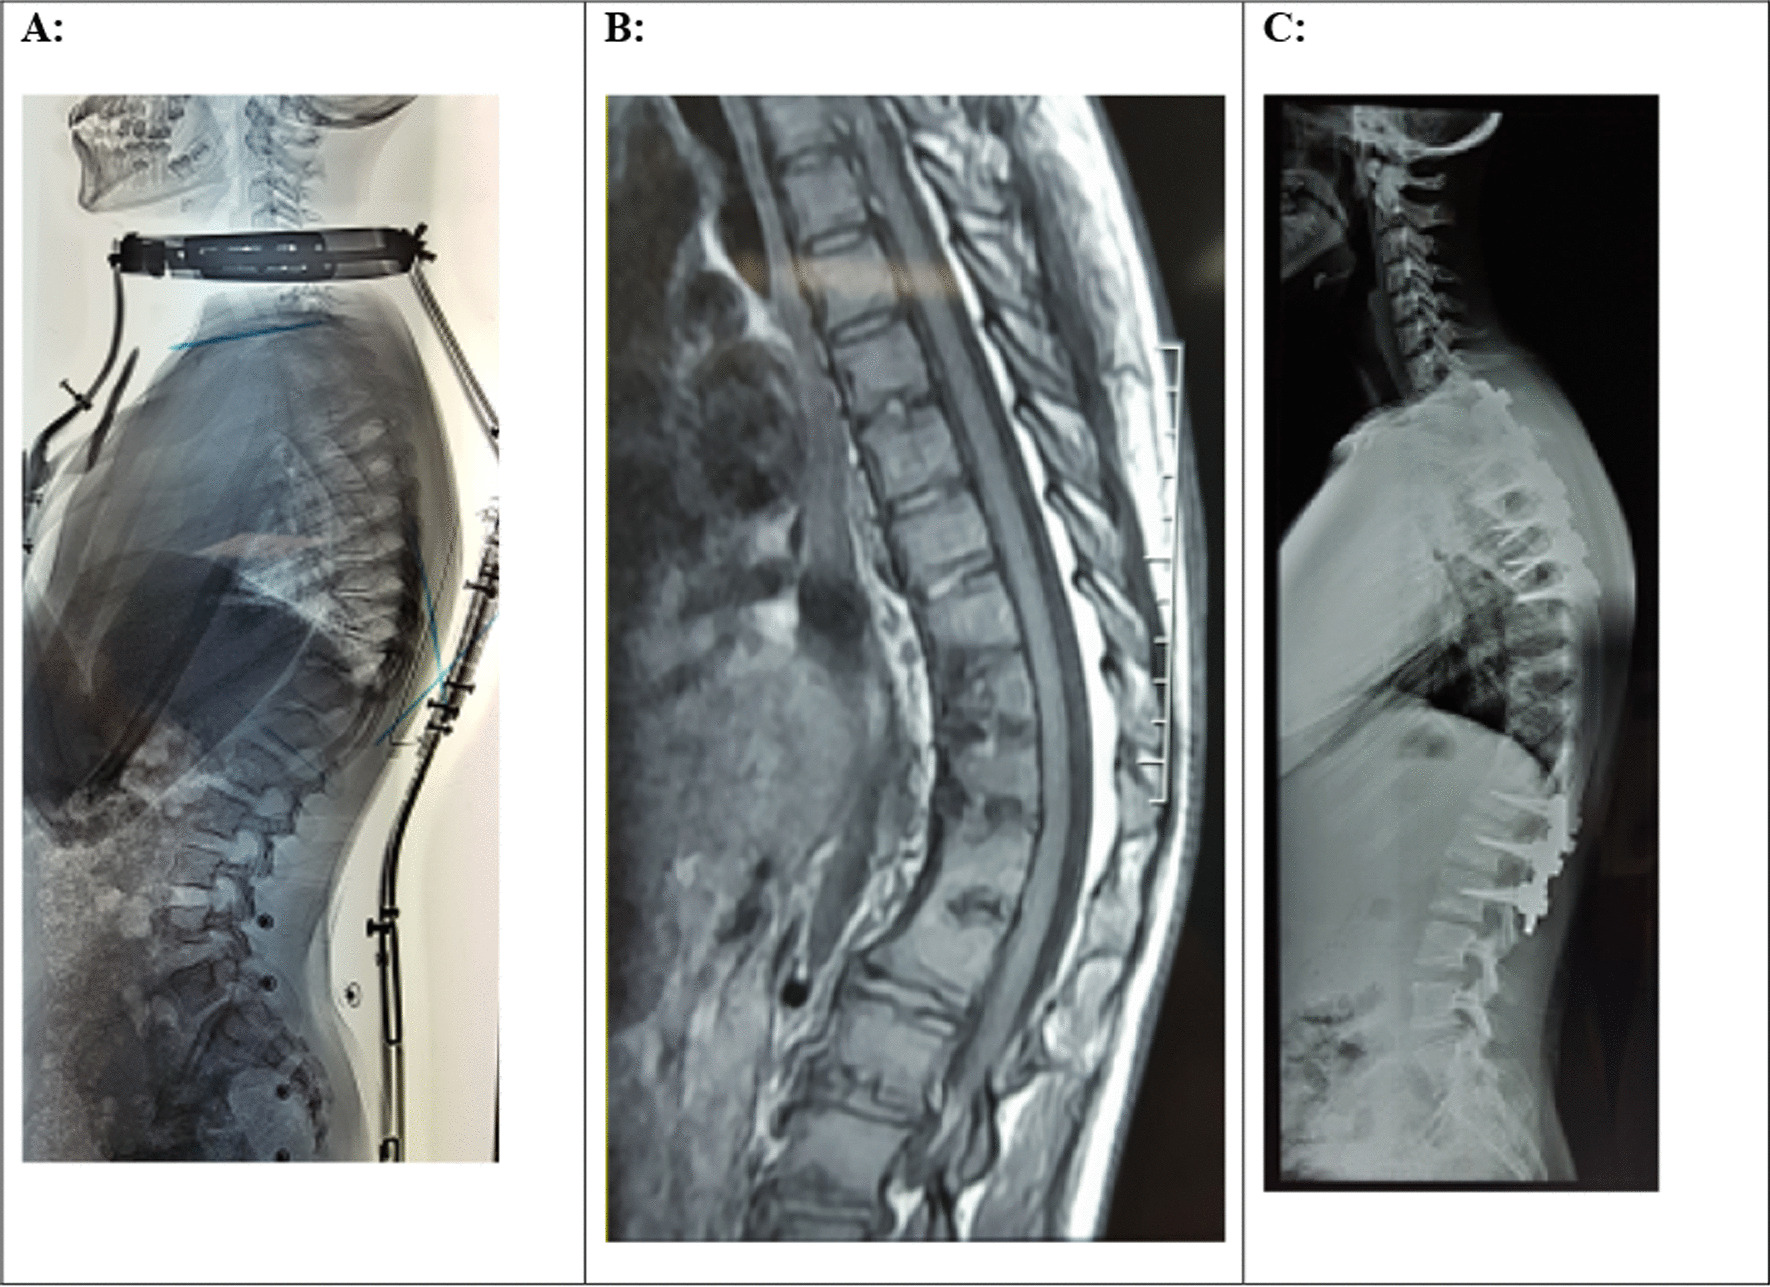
A preoperative lateral radiograph of the spine. There is narrowing noted at the T8/12 disc spaces anteriorly. B MR imaging with sagittal T1 sequences through the thoracolumbar spine demonstrate loss of anterior disc height and fusion at the T8/12 levels. C 5-year post-operative lateral radiograph of the spine
